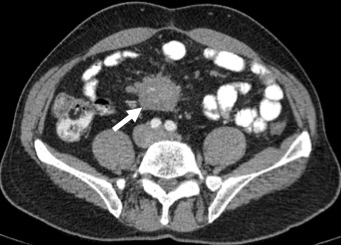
Axial CT scan with contrast, demonstrating a heterogenous soft tissue mass with calcifications and lymphadenopathy
